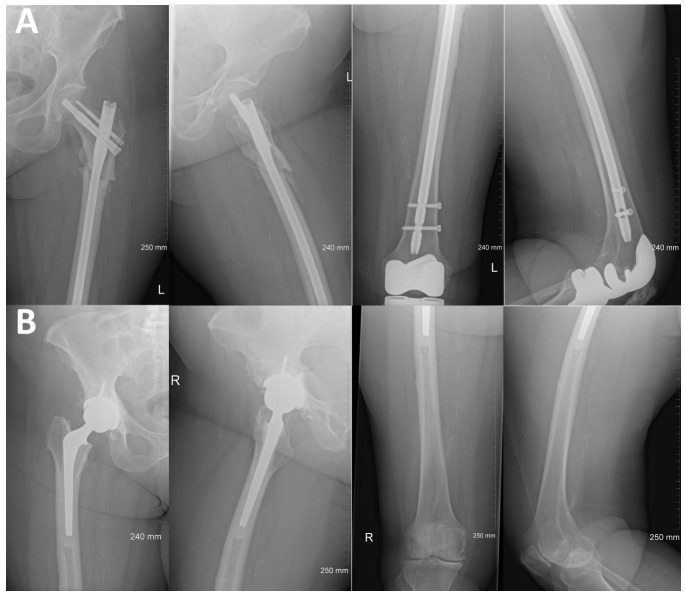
(A)—Three-month postoperative radiographs of left femur demonstrating delayed healing of subtrochanteric fracture with stable intramedullary nail fixation (top). (B)—Radiographs of the contralateral femur demonstrating lateral cortical beaking and thickening distal to the previous hip prosthesis (bottom).
